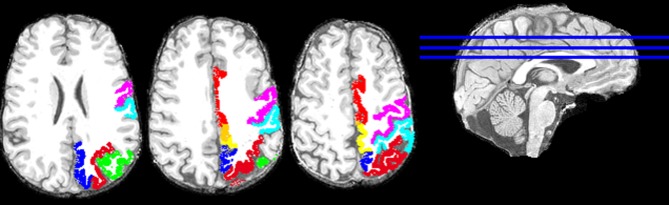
Areas of the fronto-parietal cluster, enclosed in the blue box in Figure 1, are plotted on the T1-weighted structural images of one subject (left hemisphere). Three axial slices are shown. Cortical areas of the left hemisphere are presented with the following colors: red, Cingulate Gyrus; violet, Precentral Gyrus; cyan, Postcentral Gyrus; yellow, Paracentral Lobule; green, left Inferior Parietal Lobule; blue, left Precuneus; and dark red, left Superior Parietal Lobule.
